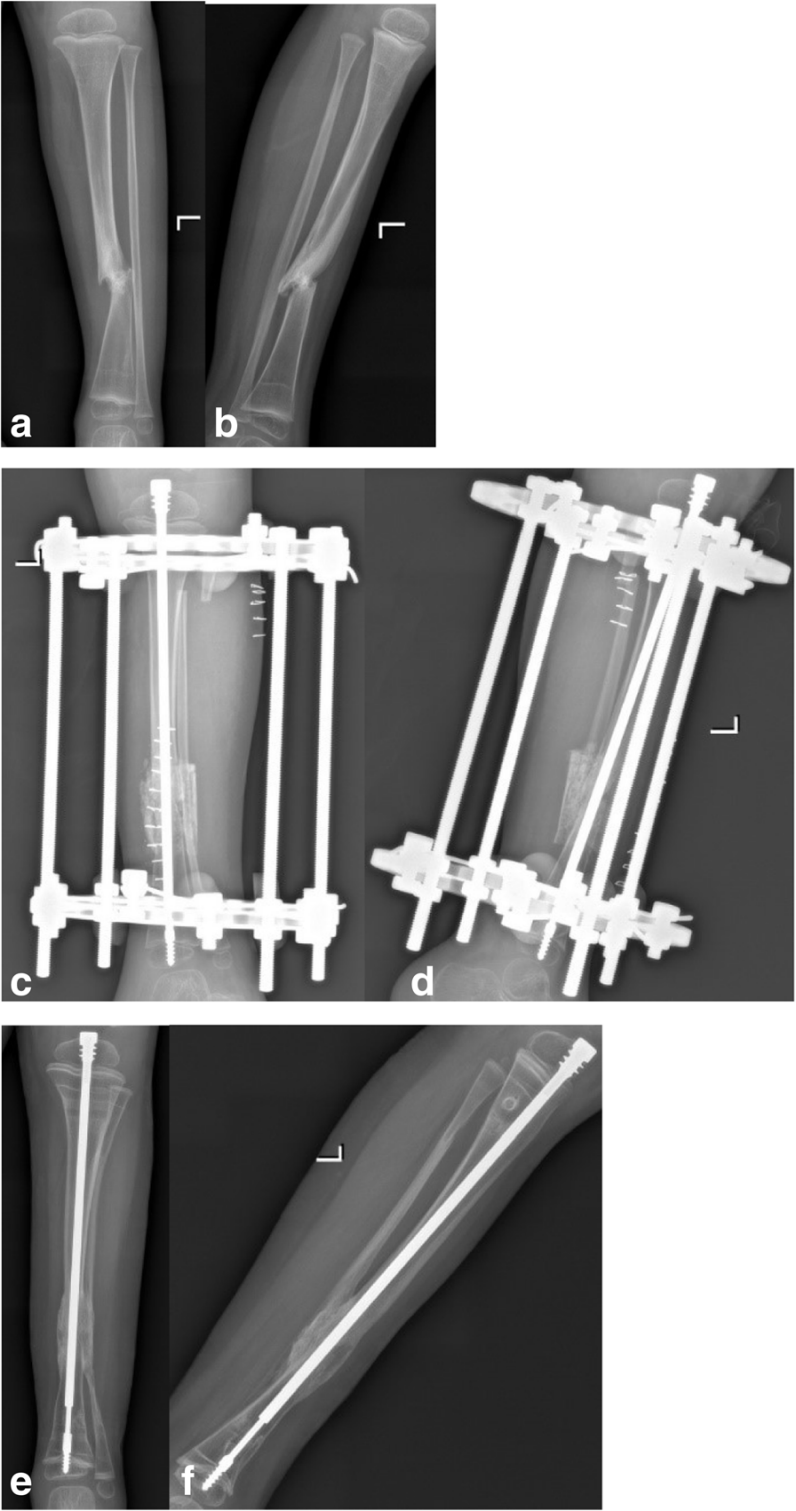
a, b A 4-year-old boy. Preoperative anteroposterior and lateral radiographs. c, d The X-ray image at 1 week post-operation. e, f Twelve months after the operation
